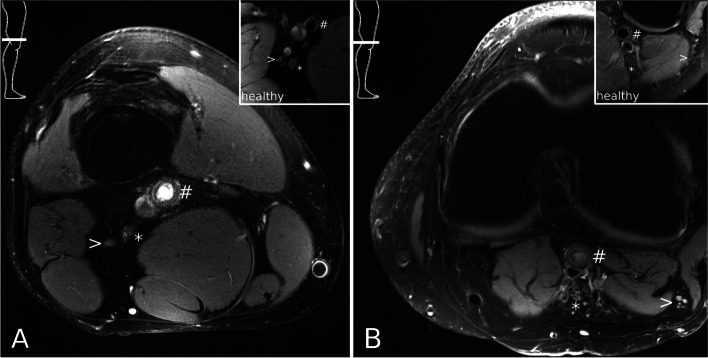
MR Neurography of the popliteal region. Images were acquired at 3 T (Siemens Magnetom SkyraFit, Siemens Healthineers, Erlangen, Germany) using a high resolution, T2-weighted, fat-saturated turbo spin-echo sequence (TR 6000 ms, TE 30 ms, FA 180°, 3 mm slice thickness, FoV 130 mm, matrix size 512). > peroneal nerve, * tibial nerve, # popliteal artery. The smaller images on the upper right part depict the morphology of the nerves and vessels in an age-matched healthy volunteer. A Patient 1. Note some hyperintense nerve fascicle bundles in the tibial nerve, and the missing "flow void" of the popliteal artery. B Patient 2. Note multiple hyperintense and slightly enlarged nerve fascicle bundles of both tibial and peroneal nerve, and the missing "flow void" of the popliteal artery"
